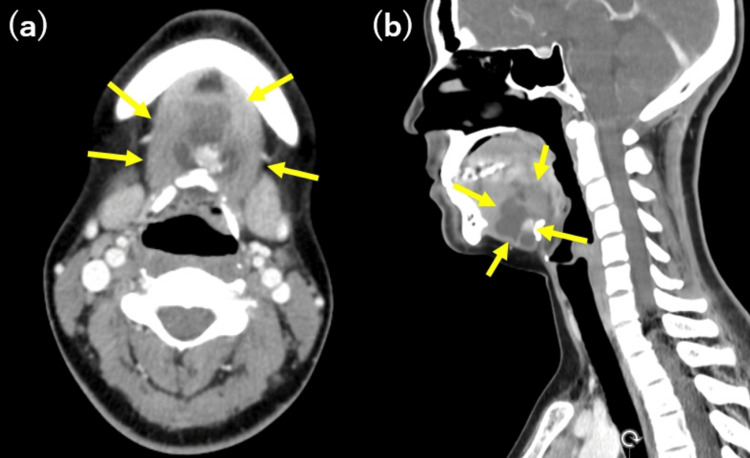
Preoperative contrast-enhanced computed tomography (CT) images (a) Axial view. (b) Sagittal view. A mass is seen anterior to the hyoid bone with internal calcifications. The images are obtained with contrast enhancement. The yellow arrow indicates the cystic component of the lesion.
